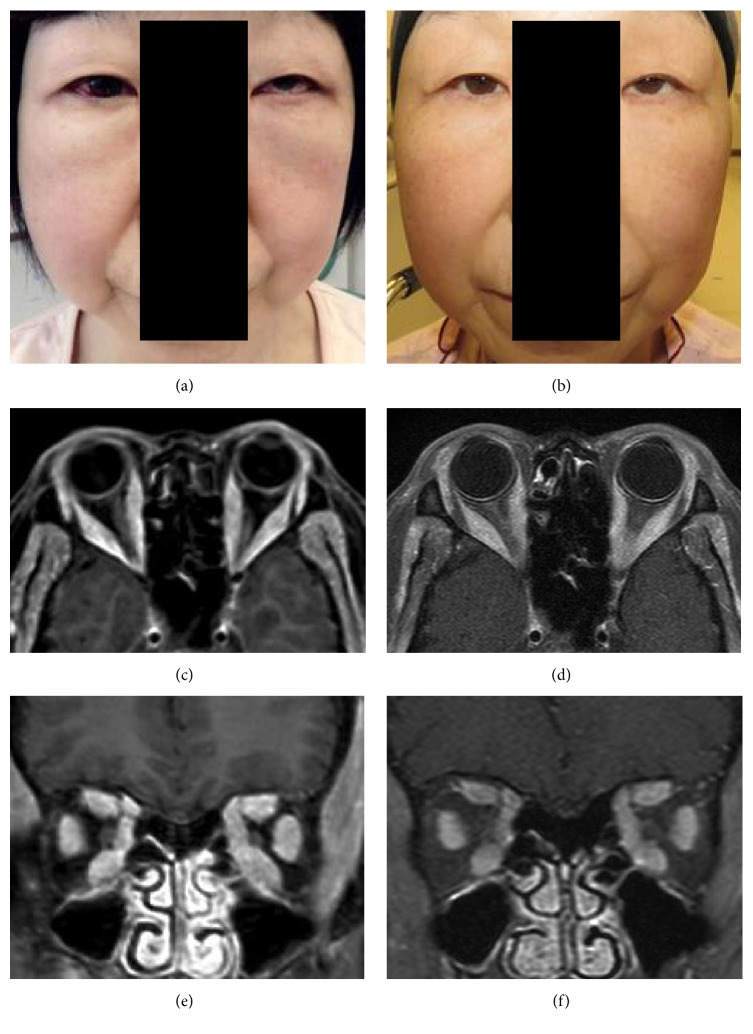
External facial photograph and enhanced magnetic resonance (MR) images with contrast of a patient diagnosed with orbital T-cell lymphoma. (a) External photograph shows moon face countenance at the initial examination. (b) External photograph shows relief of moon face countenance 1.5 months after aggressive chemotherapy with alternate hyper CVAD therapy (cyclophosphamide, vincristine, adriamycin, and dexamethasone), and MA therapy (methotrexate and cytarabine) was performed. (c, e) MR images showing an enlargement of all of the extraocular muscles at presentation. (d, f) MR images showing an attenuation of such findings at 1.5 months during the chemotherapy.
